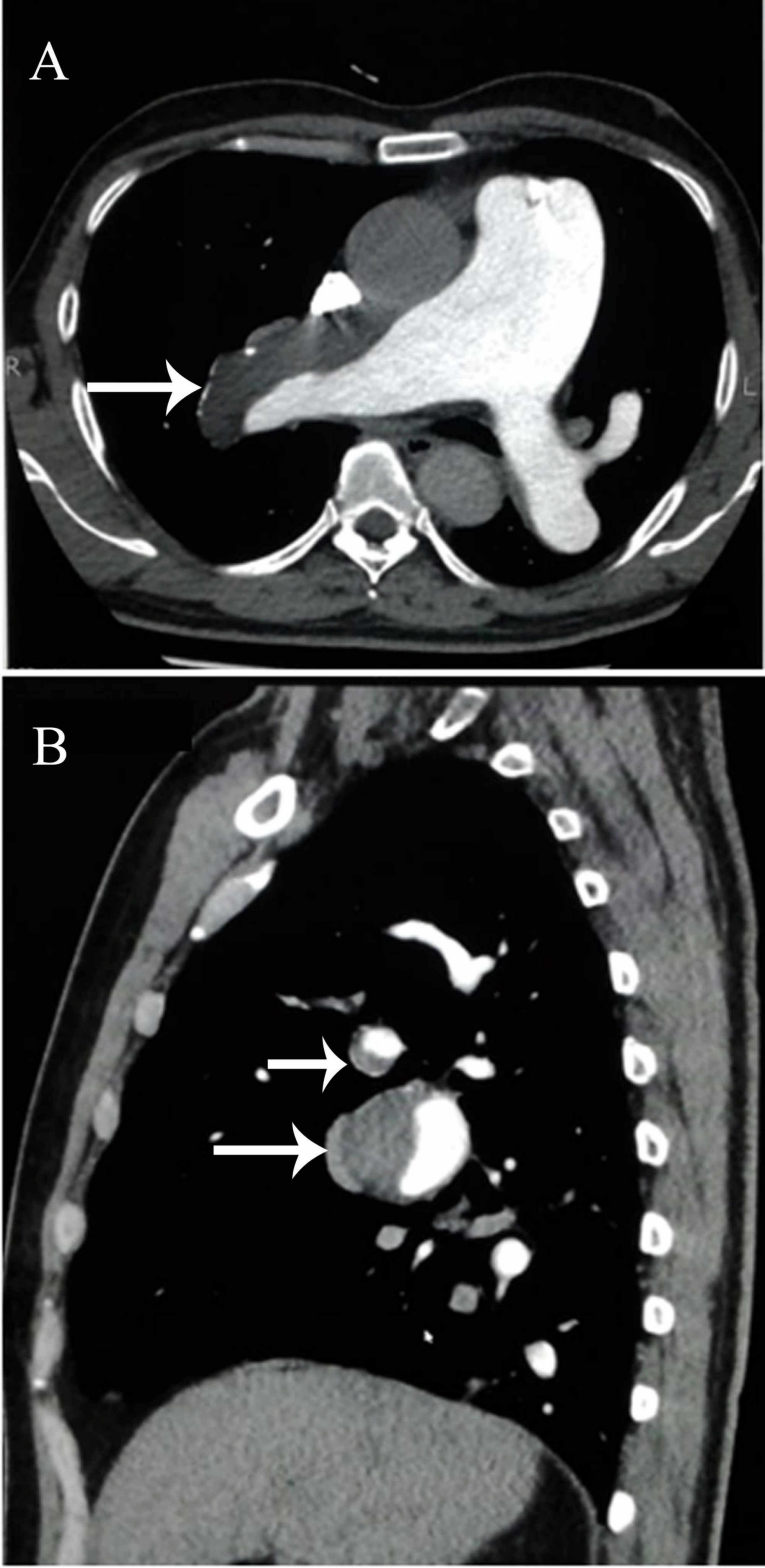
Computed Tomography Scan Chest Axial section (A) and sagittal section (B) through the thorax: An eccentric filling defect is seen in right main pulmonary artery also involving the segmental branches of lower lobe suggestive of a thrombus (arrows). There is associated dilatation of the main pulmonary trunk.
